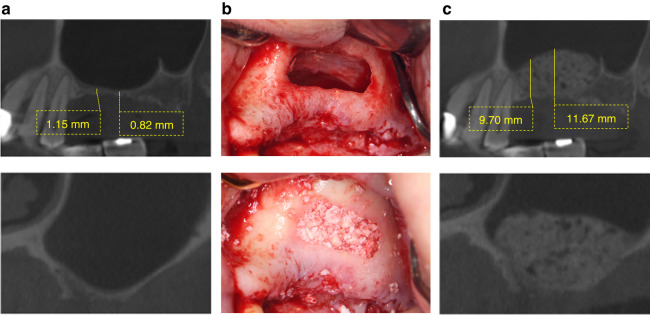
Case 2: a Pre-operative CBCT assessment, b surgical procedure, and c post-operative CBCT assessment of patient in case 2
